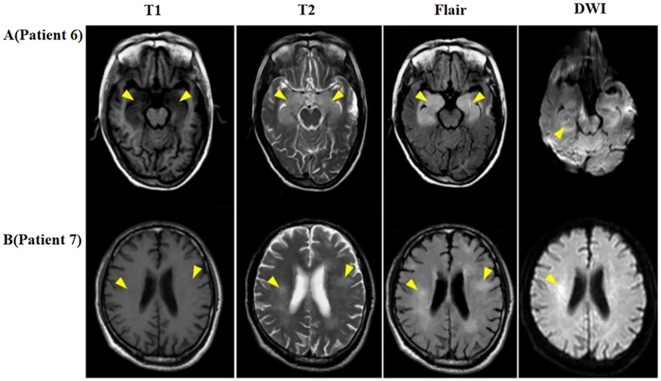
Axial brain MRI findings in two patients with anti-mGluR5 encephalitis. (A) Patient 6: Bilateral hippocampal lesions showing hypointensity on T1-weighted images, hyperintensity on T2-weighted and fluid-attenuated inversion recovery (FLAIR) sequences, and subtle restricted diffusion on diffusion-weighted imaging (DWI) (yellow arrowheads). (B) Patient 7: Bilateral white matter lesions displaying hypointensity on T1-weighted images, hyperintensity on T2-weighted/FLAIR sequences, and subtle restricted diffusion on DWI (yellow arrowheads). MRI, magnetic resonance imaging; FLAIR, fluid-attenuated inversion recovery; DWI, diffusion-weighted imaging.
